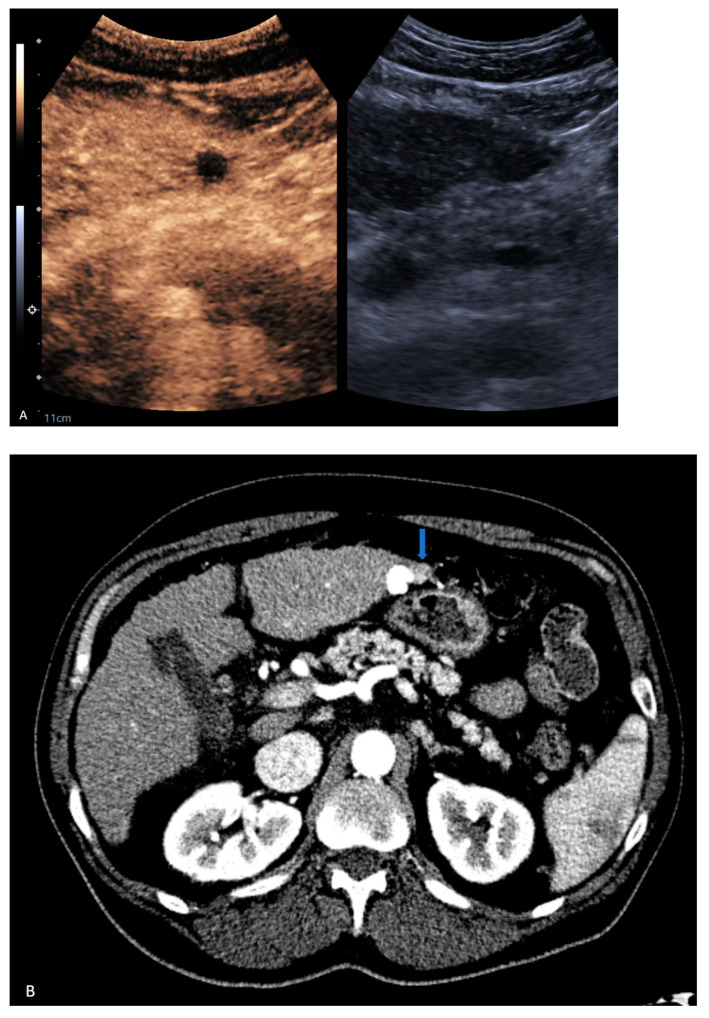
A 68-year-old male with cirrhosis secondary to MASH. (A) CEUS and accompanying grayscale demonstrate the completely treated lesion. Initial CT (B) shows region of enhancement (blue arrow) adjacent to the Ethiodol that was called residual disease. Three-month follow-up CT showed region of enhancement was perfusional and not viable tumor.
